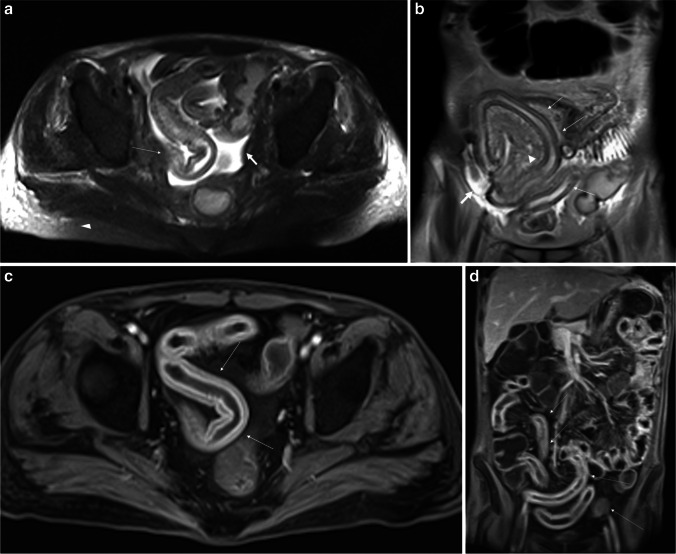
Acute intestinal GVHD in 36-year-old male, Glucksberg grade II (moderate clinical grade). The MRI severity score is 20 and considered as moderate-to-severe disease. The entire ileum is involved. a (T2-weighted fat-suppressed axial image) and b (T2-weighted coronal image) show diffuse continuous *edema with stratification of the bowel wall (thin arrows)*, mild ascites (thick arrows), and edema of the mesenteric fat tissue and of declivous tissues (arrowheads). c (T1-weighted gadolinium-enhanced axial image) and d (T1-weighted gadolinium-enhanced coronal image) show marked wall contrast enhancement and wall stratification (thin arrows). All the pictures clearly show the diffuse *stiffness and *continuous involvement* of the bowel loops (thin arrows). Intestinal stiffness*: intended as a continuous diffuse and circumferential intestinal wall edema (continuous wall thickening) which causes a serpiginous and rigid appearance of the involved bowel, showing smooth rather than sharp angles
